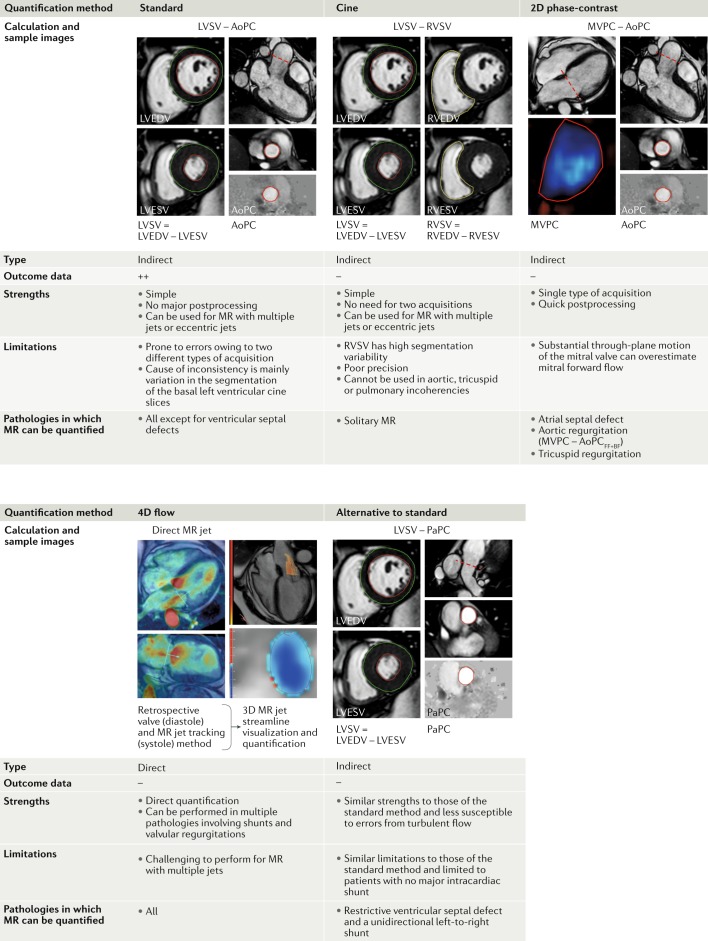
Main methods of MR quantification by cardiovascular magnetic resonance imaging. Prognostic and diagnostic outcome data are most available for the standard method of quantifying mitral regurgitation (MR) volume (MR_vol_), which is left ventricular stroke volume (LVSV) minus aortic phase-contrast forward volume (AoPC). Other methods have particular advantages or disadvantages. In routine clinical practice, cross-checking between methods is recommended. FF+BF, forward flow plus backward flow; LVEDV, left ventricular end-diastolic volume; LVESV, left ventricular end-systolic volume; MVPC, mitral valve phase-contrast stroke volume; PaPC, pulmonary artery phase-contrast stroke volume; RVEDV, right ventricular end-diastolic volume; RVESV, right ventricular end-systolic volume; RVSV, right ventricular stroke volume.
